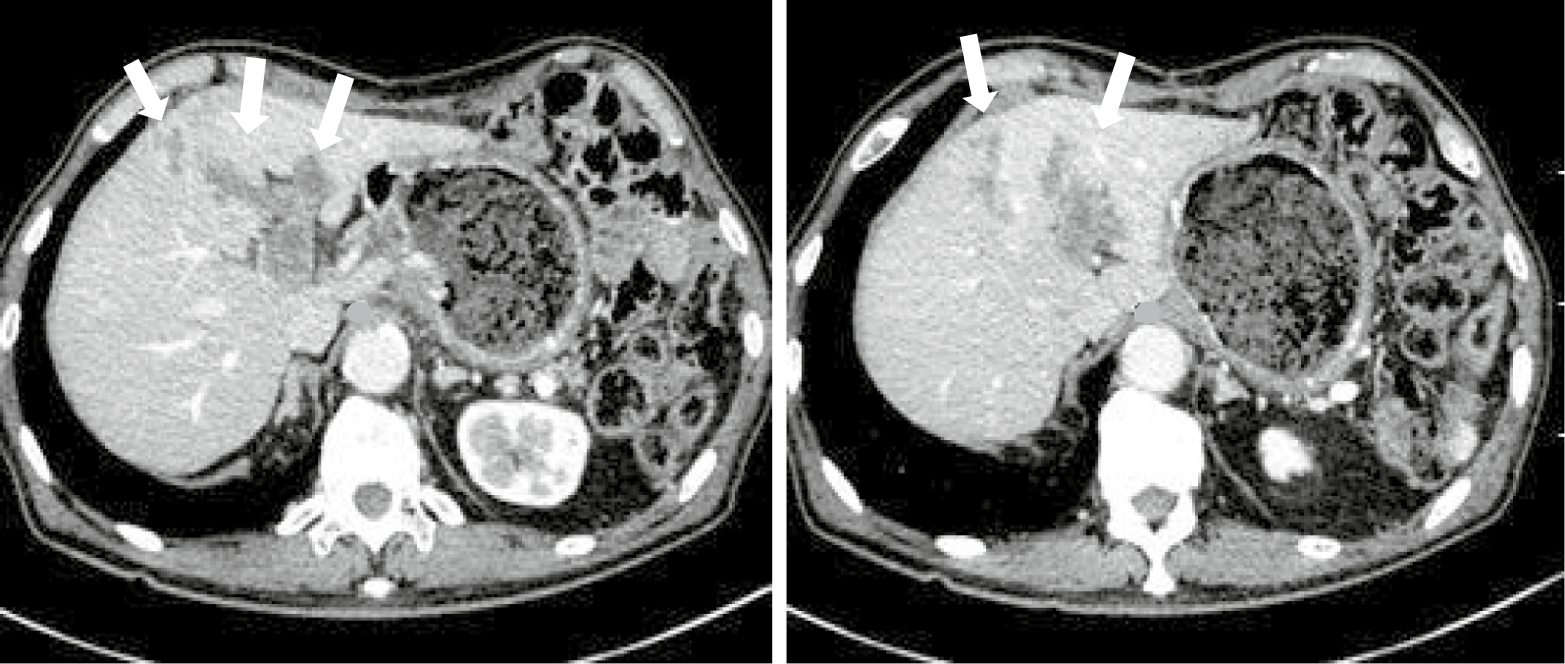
Abdominal enhanced CT of 6 months after surgery: metastatic lesions are indicated by arrows
